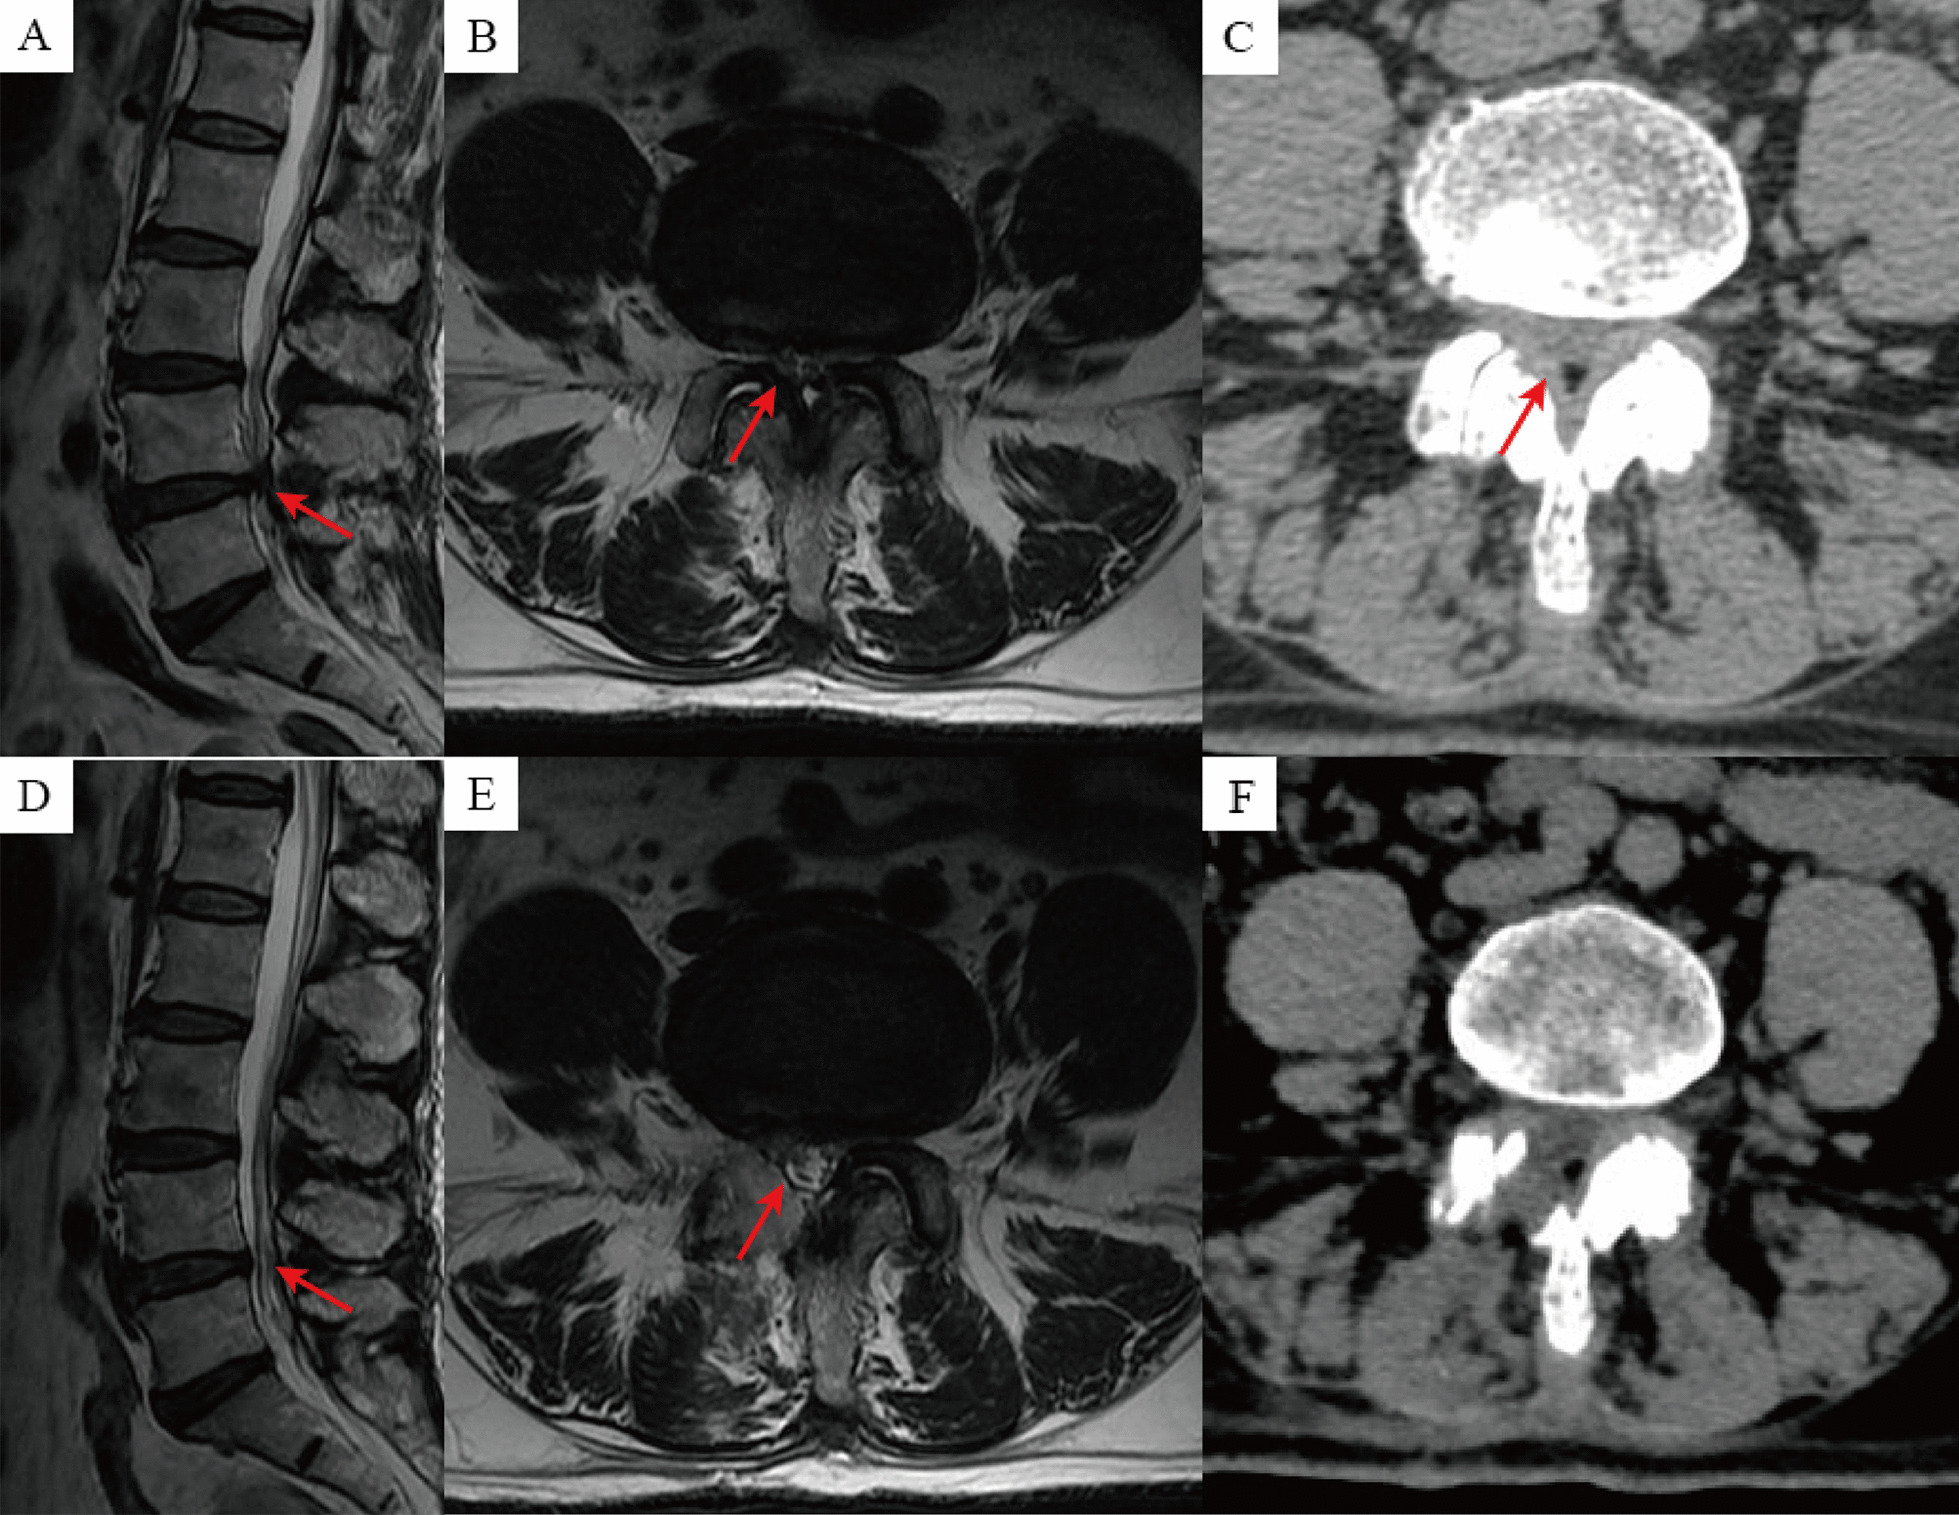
Images from a patient treated with UBE technique. A–C Preoperative MRI and CT showed L4-5 segment disc herniation with spinal stenosis; D–F Postoperative MRI and CT showed removal of the disc herniation, and adequate decompression of the spinal canal
